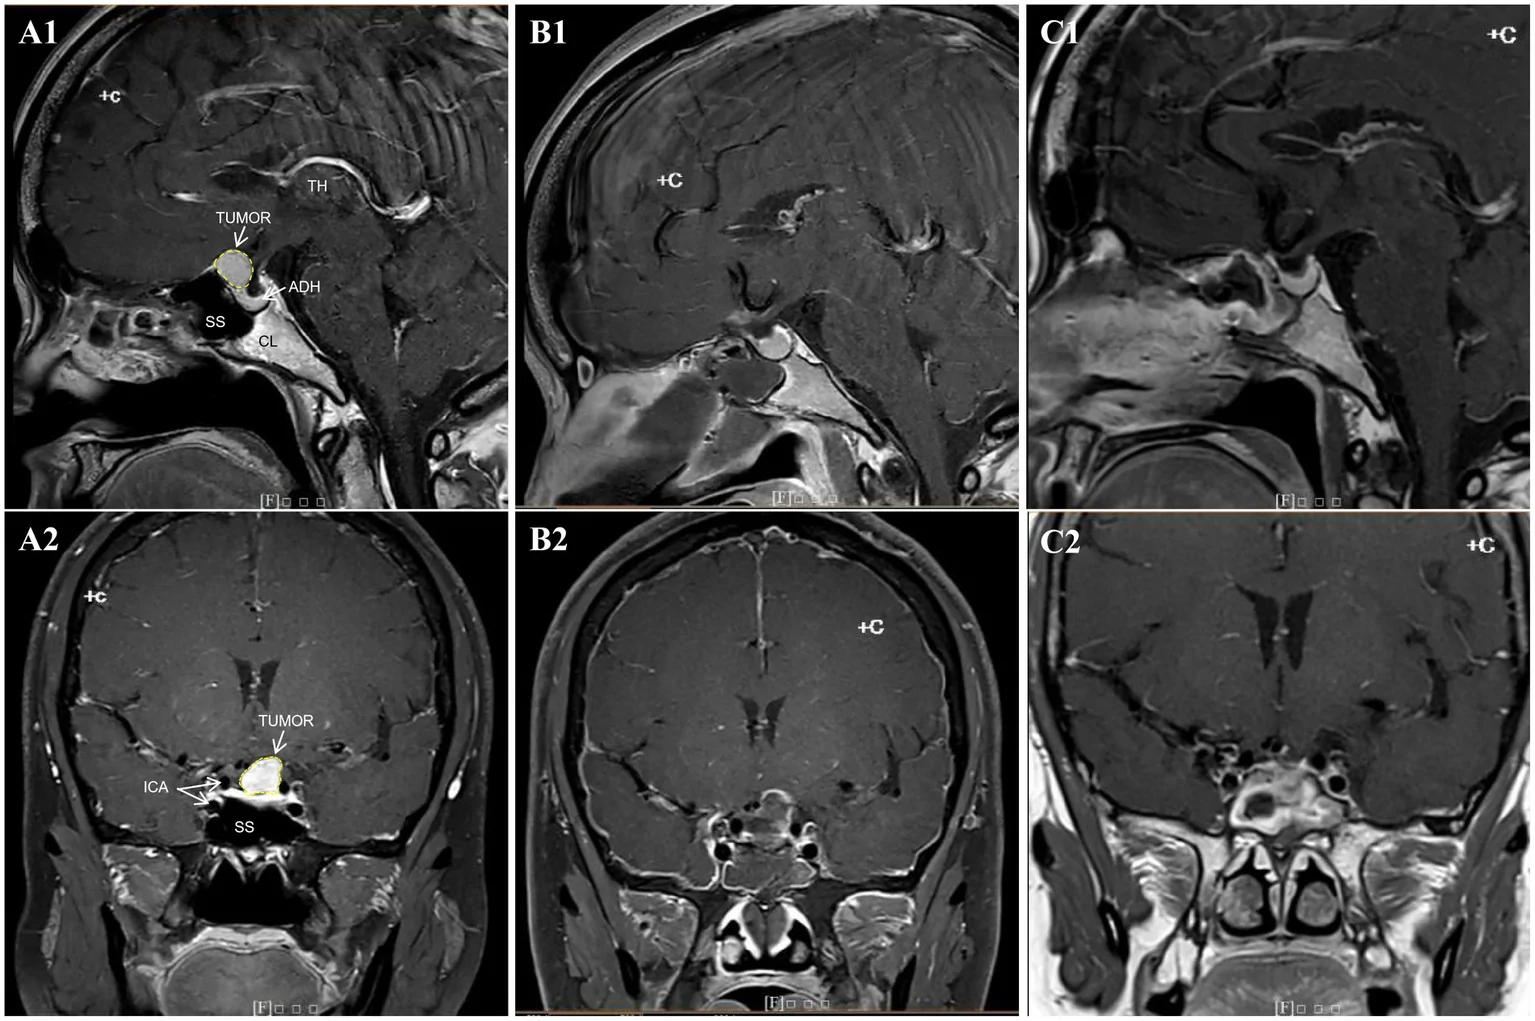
Preoperative, postoperative, and 12-month follow-up magnetic resonance images (MRI) of Patient 1. (A) Preoperative sagittal and coronal contrast-enhanced MRI. (B) Postoperative sagittal and coronal MRI confirming gross total resection. (C) Twelve-month follow-up MRI showing no tumor recurrence. TH, thalamus; ADH, adenohypophysis; SS, sphenoid sinus; CL, clivus; ICA, internal carotid artery.
